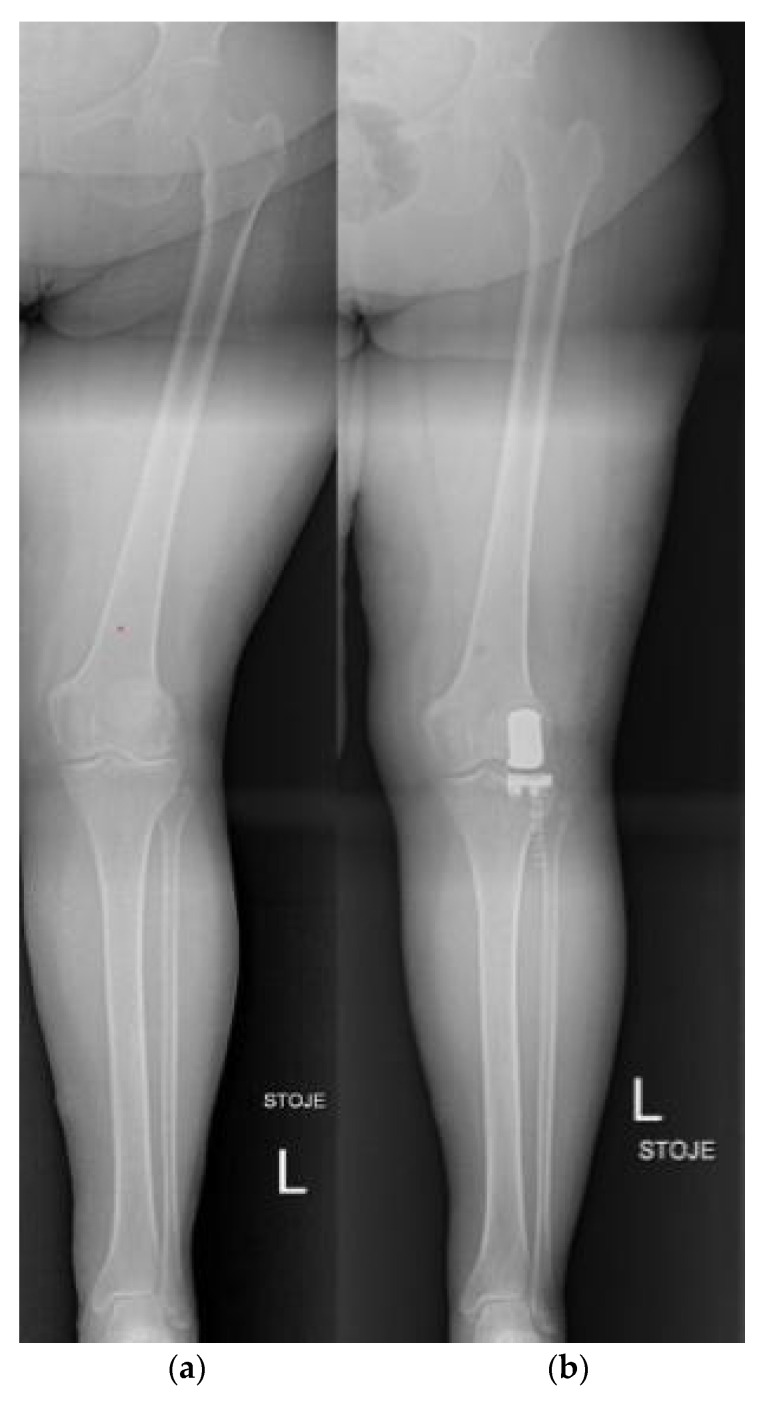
Unicompartmental (partial) knee arthroplasty (UKA). (a) preoperative X-ray; (b) postoperative X-ray after unicompartmental knee arthroplasty for isolated knee osteoarthritis of the lateral compartment.
